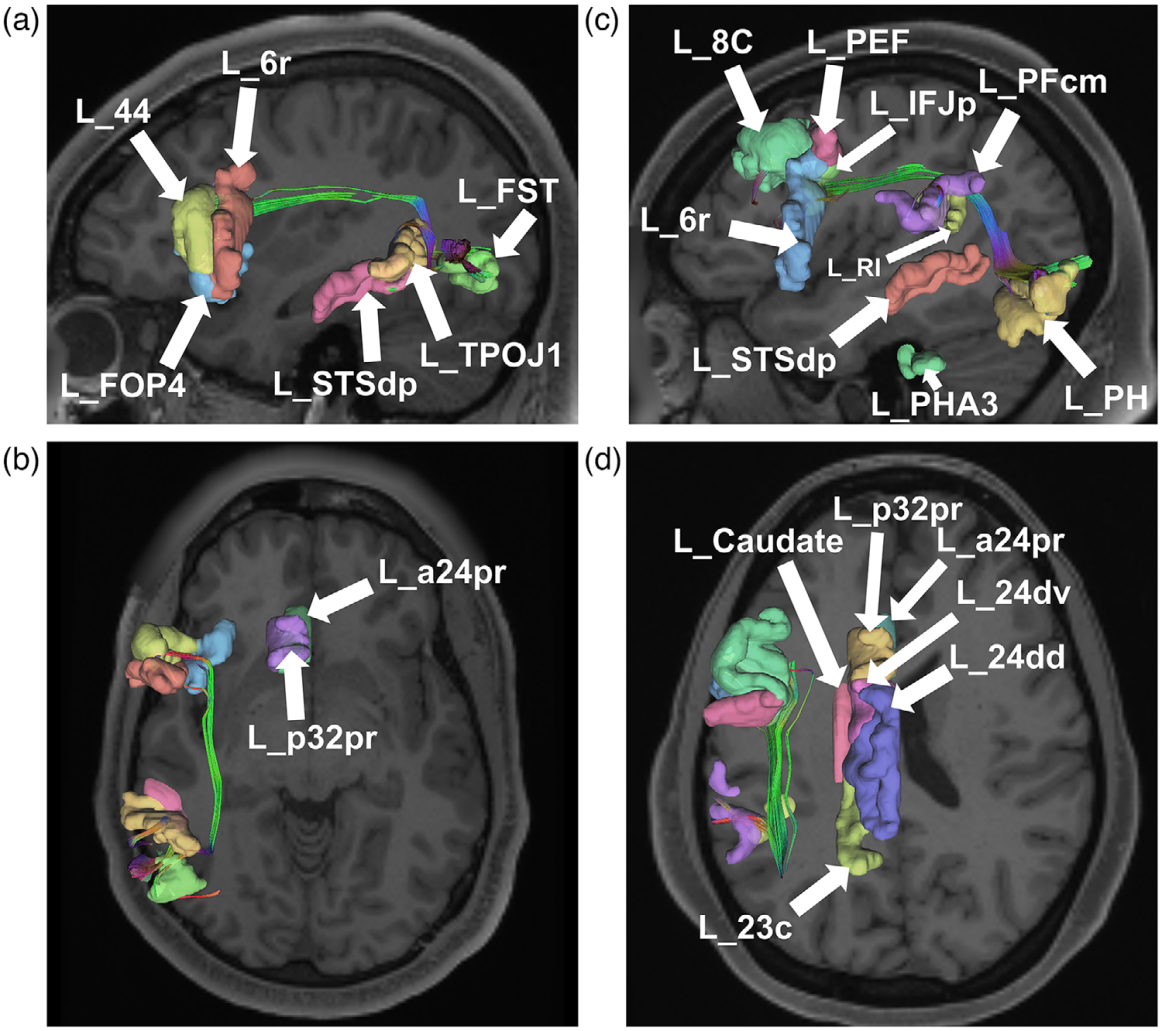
Tractographic model of areas fundus of the superior temporal sulcus (a, b) and parietal area H (c, d) and their functionally coactivated parcellations as shown on T1‐weighted magnetic resonance images in the left (L) cerebral hemisphere in sagittal and axial slices. Tractography presented is from a single healthy individual. Note that the figures are for visualization only and include parallax error. 8c, Brodmann area 8 component C; PEF, prefrontal eye field; IFJp, inferior frontal junction area/posterior; 6r, rostral premotor area 6; 44, Brodmann area 44; FOP4, frontal operculum 4; p32pr, posterior 32 prime; a32pr, anterior 32 prime; a24pr, anterior 24 prime; 24dv, ventral area 24d; 24dd, dorsal area 24d; 23c, Brodmann area 23 component C; 23d, Brodmann area 23 component D; PFcm, Brodmann area 40 PFcm complex; RI, retroinsular; STSdp, superior temporal sulcus dorsal posterior; PHA2, parahippocampal area 2; PHA3, parahippocampal area 3; TPOJ1, temporo–parieto–occipital junction 1; FST, fundus of the superior temporal sulcus; PH, parietal area H.
